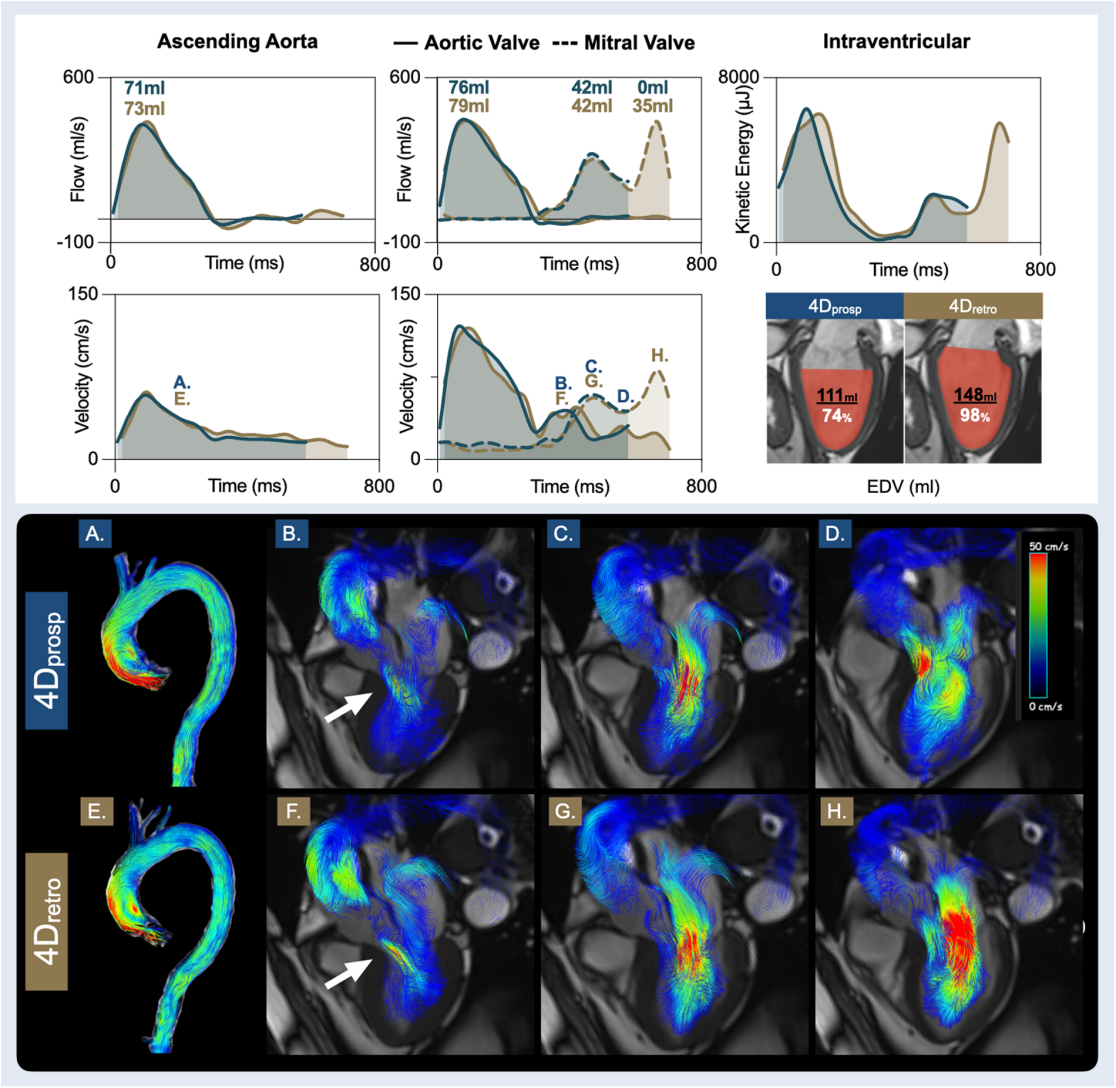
Patient with diastolic dysfunction. Presented are measurements from the prospective gated (4D_prosp_, blue, 80% cardiac cycle), and retrospective gated (4D_retro_, gold, 95% cardiac cycle) 4D flow of a 74-year-old male, with hypertension, known CAD and recent abdominal aortic repair surgery. A clinical echocardiography report diagnosed diastolic dysfunction grade I, light aortic valve regurgitation with an asymptomatic ascending aortic dilation (40 mm) and LV hypertrophy. The bottom two rows display 4D flow streamlines colour coded by velocity. The time point of each image (A–F) is labelled on the velocity graphs. It can be observed that despite an abnormal aorta (A/E), ascending aortic and aortic valve volumes, flows and velocities are similar during systole, and both depict the aortic valve regurgitation (B/F) occurring during diastole. Both sequences yielded similar findings for early diastole. Yet, due to the diastolic dysfunction, a significant portion of diastolic filling occurs in the final 150 ms that was not captured by prospective gating. As a result, it can be observed in the final phase captured by 4D_prosp_ (D), the ventricle is still filling. Consequently, neither true peak A-flow rate nor A-velocity was quantified. In image H with 4D_retro_, a higher A-flow rate and A-velocity was present in late diastole than early diastole (G), therefore, total diastolic transmitral volume, flow and end-diastolic volume (EDV) are underestimated.
